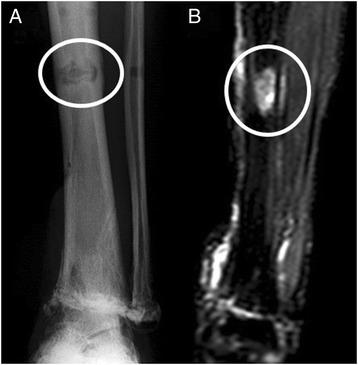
Anteroposterior view of an osteolytic lesion. Plain radiography shows an osteolytic lesion in the tibial pin tract (a). T2-weighted magnetic resonance imaging shows fluid collection (b). The circle shows osteolytic lesion (a), and fluid collection (b), respectively
